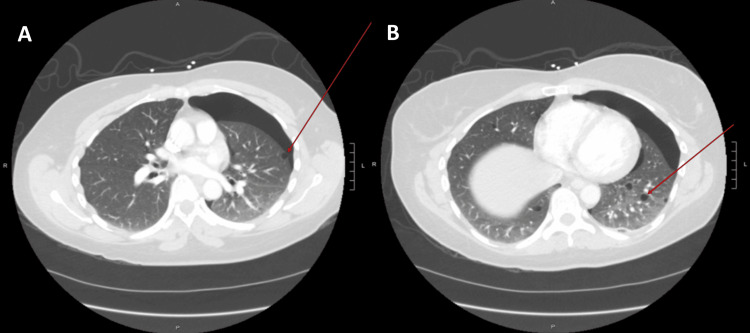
High-resolution CT chest (axial view) showing (A) the ruptured bleb in the left upper lobe, and (B) multiple bilateral cystic lesions in the lung parenchyma.
